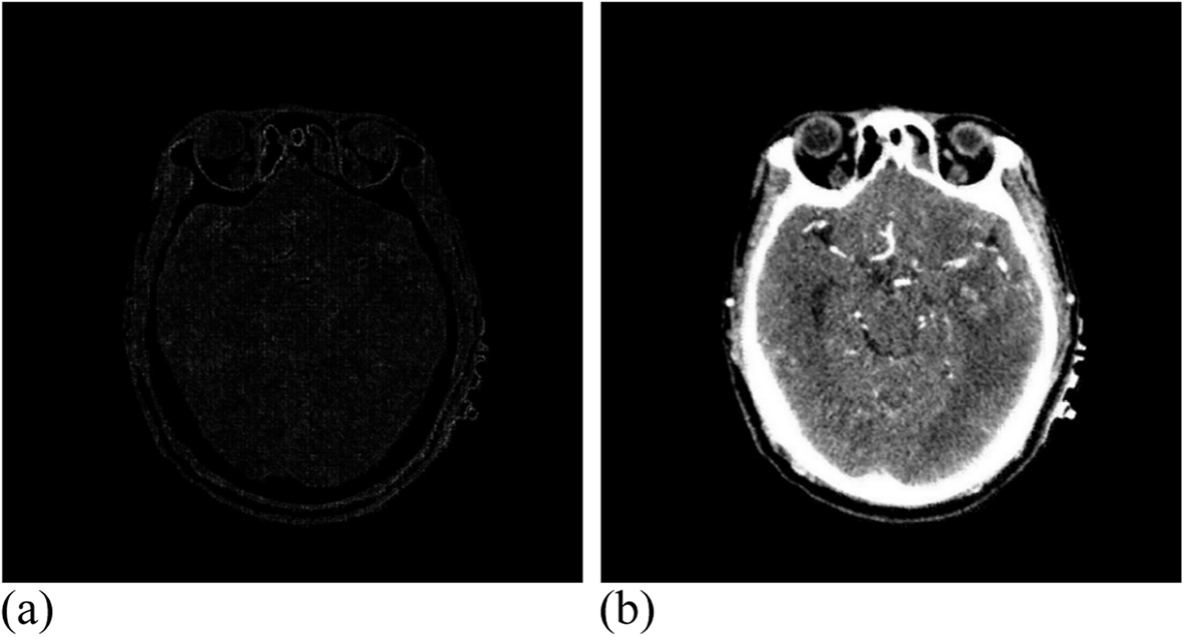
CT data after registration. (a): Difference between CT reference image and registration image; (b): CT registration image
